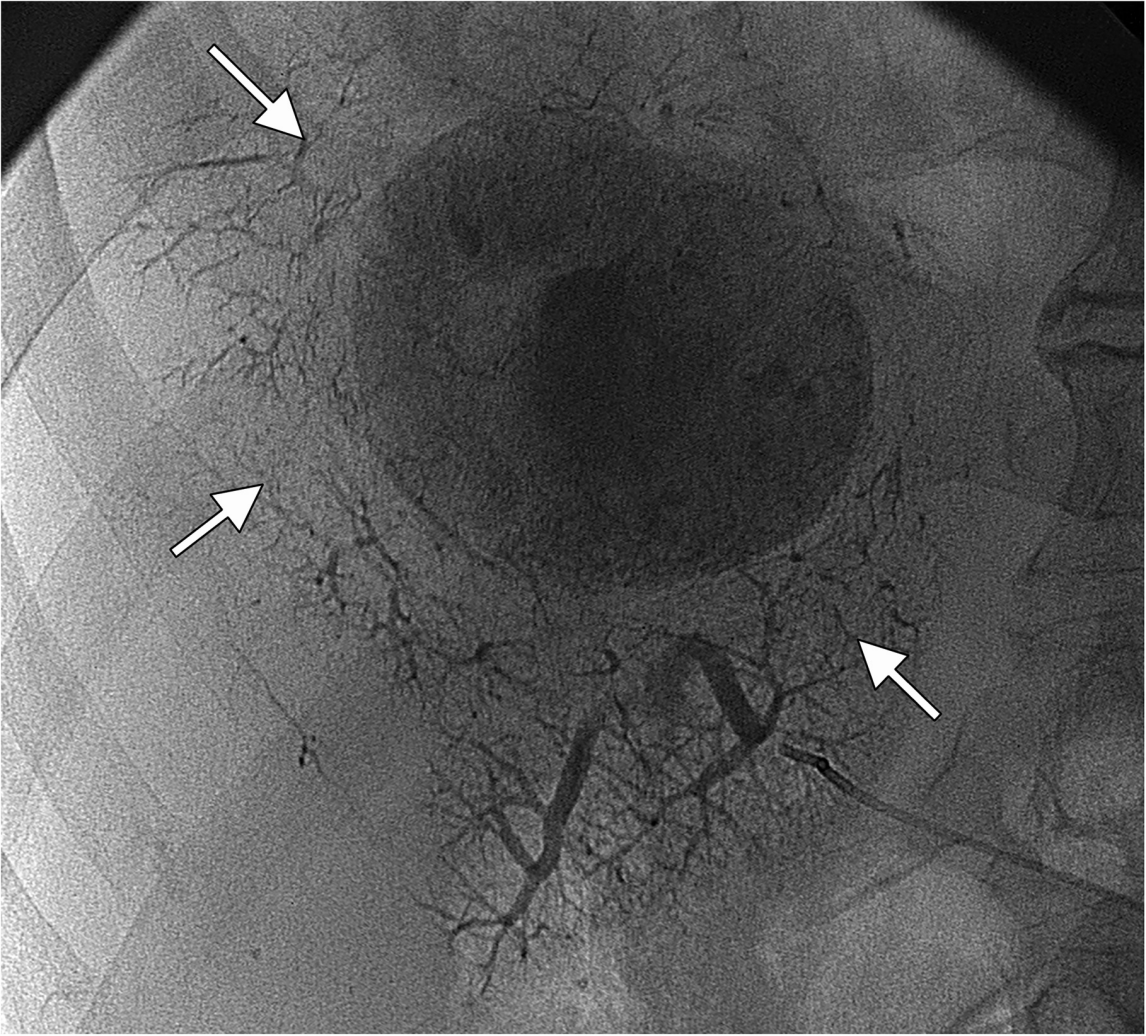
Radiographic image displaying PPLE (arrows) during TACE for HCC. In this 86-year-old man, pathological anatomical diagnosis had demonstrated a highly differentiated tumor. Complete remission was achieved, and the patient was still alive at follow-up after eight years. HCC, hepatocellular carcinoma; PPLE, peritumoral portal lipiodol enhancement; TACE, transarterial chemoembolization.
